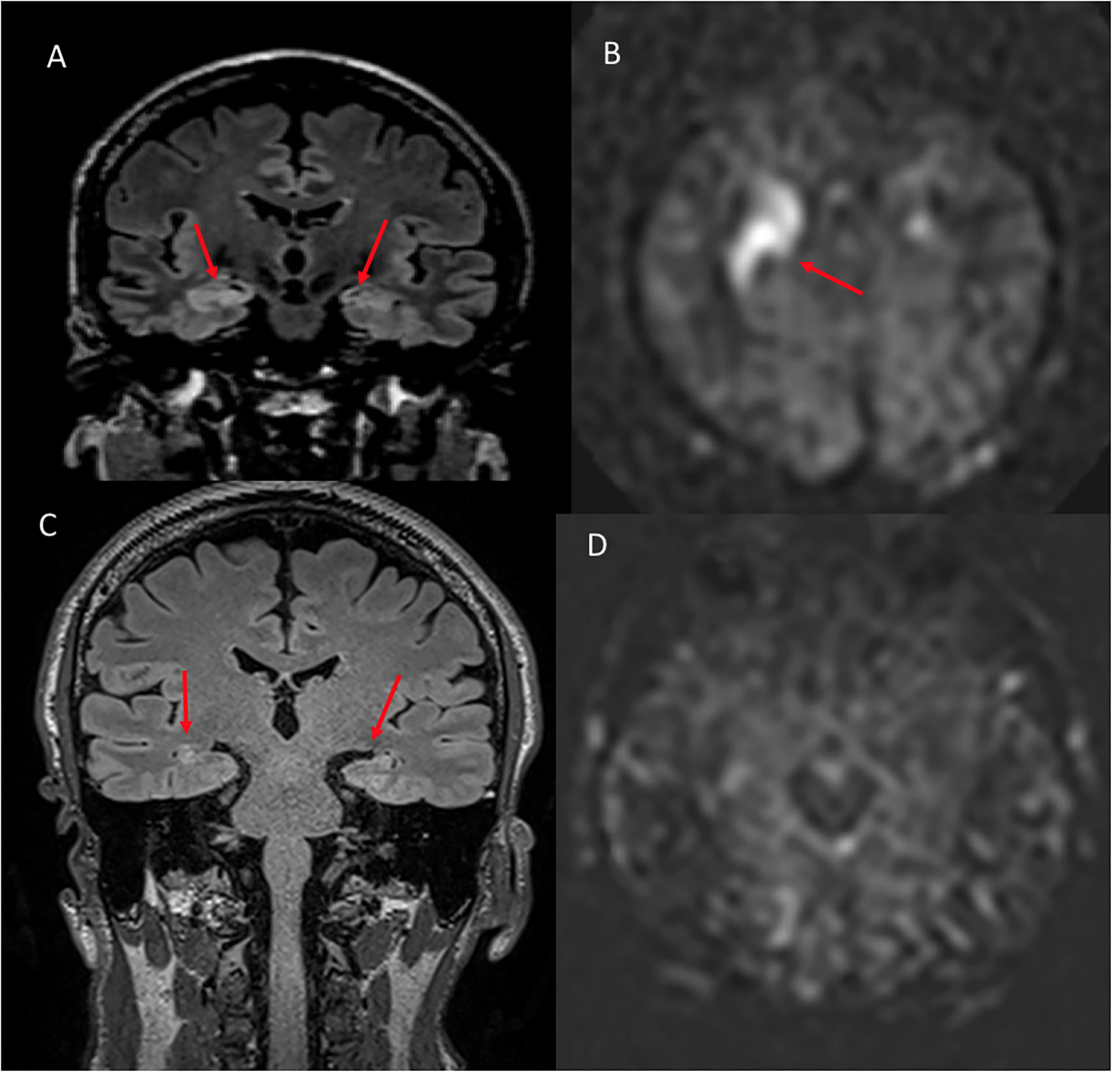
A 48 year old male with memory loss and seizures. (A) Coronal FLAIR shows hyperintense signal in the right greater than left hippocampus and amygdala. (B) ASL shows hyperperfusion in the corresponding regions. (C) Six month follow up coronal FLAIR shows hyperintense signal in the bilateral hippocampi compatible with mesial temporal sclerosis. (D) Six month follow up ASL demonstrates complete resolution of the previously seen hyperperfusion.
